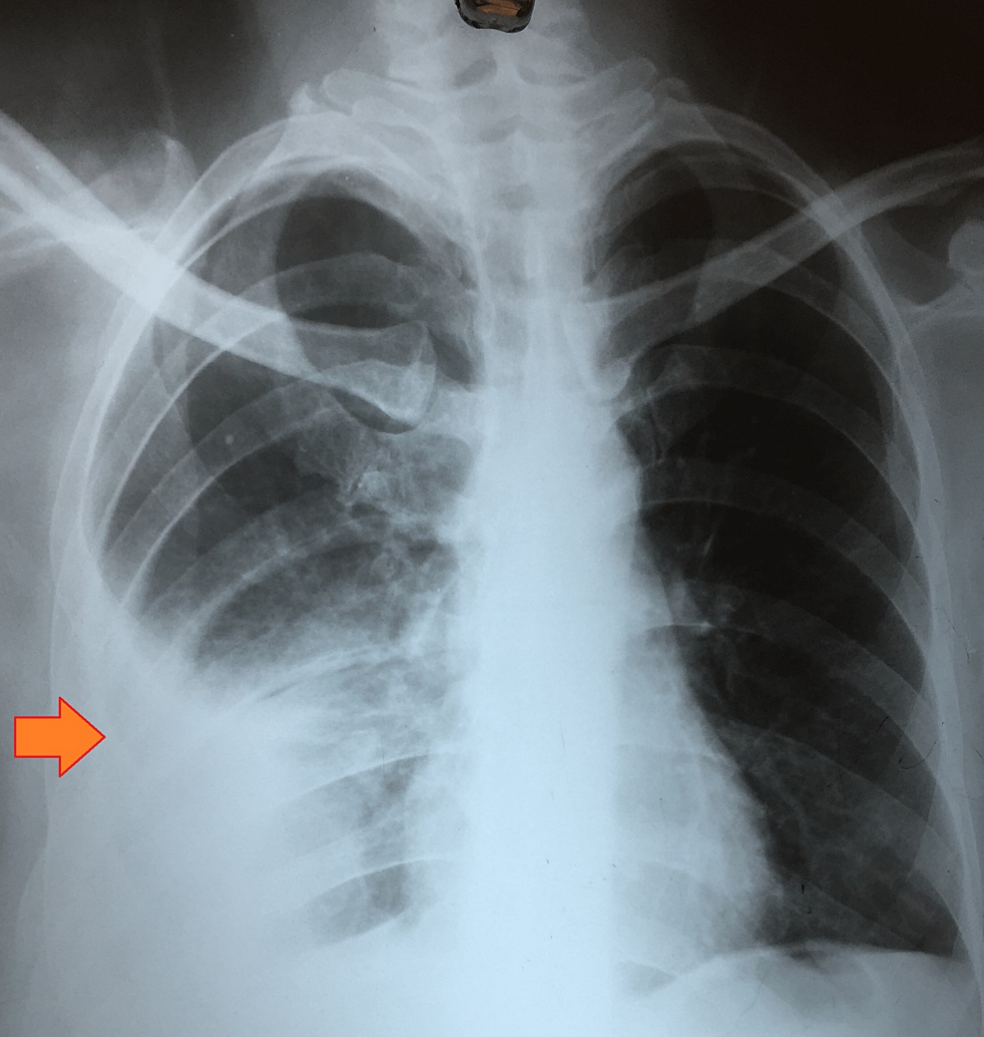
A chest radiograph (P-A) view suggestive of right-sided pleural effusion with consolidation in the right middle lobe P-A: postero-anterior
